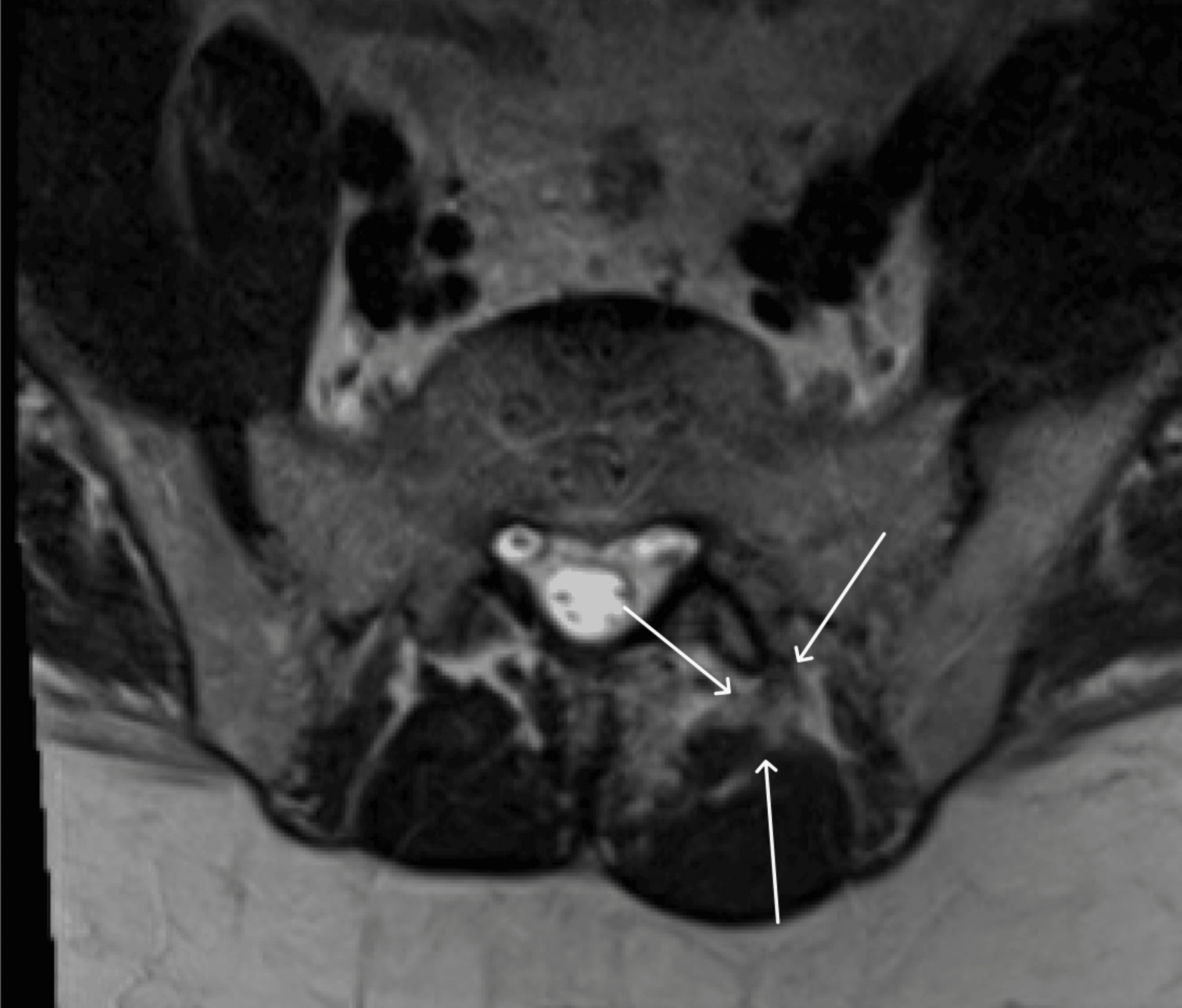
T2-Weighted MRI With Contrast in the Sacral Plane The MRI was interpreted as left paraspinal muscle edema extending from L2 to S2, largely consistent with pyomyositis in the clinical context. MRI: magnetic resonance imaging
